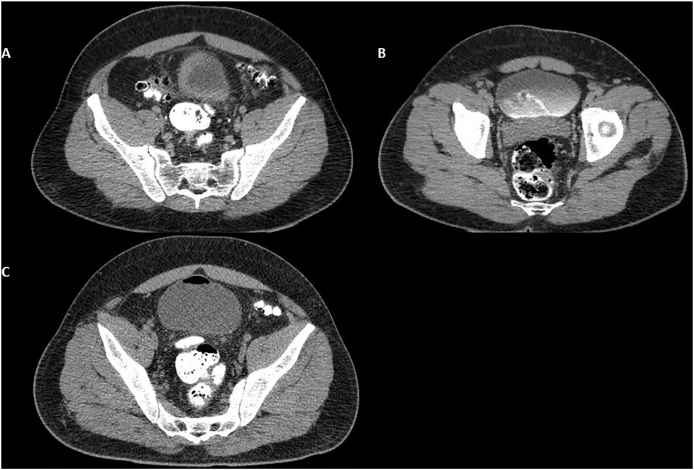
A. CT scan with oral contrast showing sigmoid colon contrasted but no evidence of contrast overflow to the bladder. B. CT scan after intravenous contrast with no evidence of communication between the urinary bladder and sigmoid colon, also it is possible to visualize the contrast flush from left ureter to the urinary bladder. C. New CT scan with oral contrast with no evidence of contrast overflow from the sigmoid colon to the urinary bladder.
